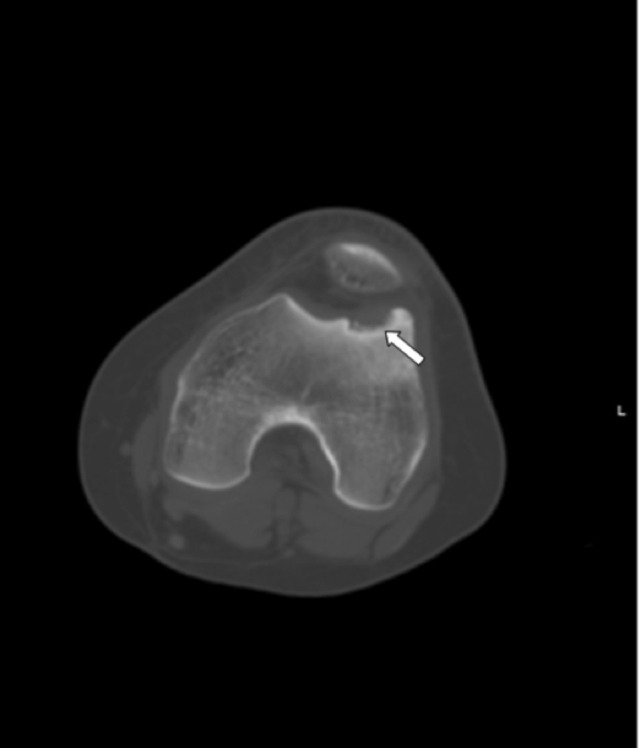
Preoperative computed tomography (CT) demonstrating lateral femoral trochlea OCD (white arrow).
